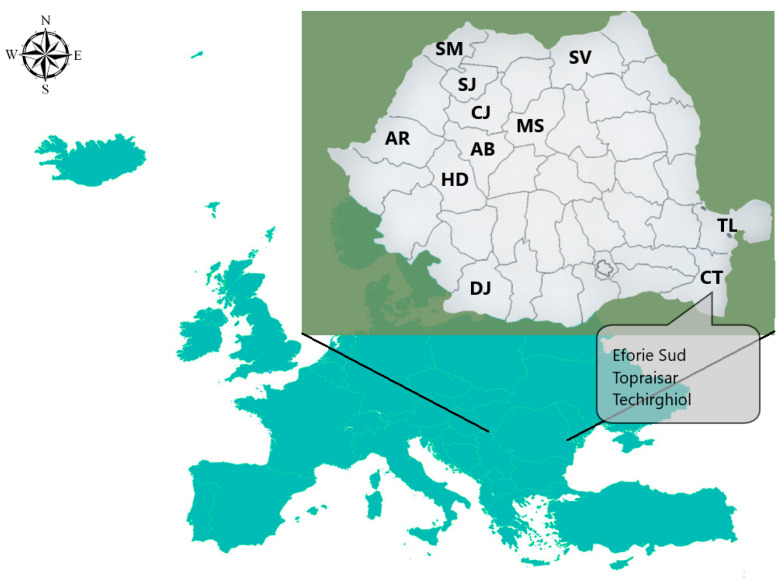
The map of the egg sample’s locations. AB, Alba County; AR, Arad County; CJ, Cluj County; CT, Constanta County; DJ, Dolj County; HD, Hunedoara County; MS, Mures County; SM, Satu Mare County; SJ, Salaj County; SV, Suceava County, TL, Tulcea County.
